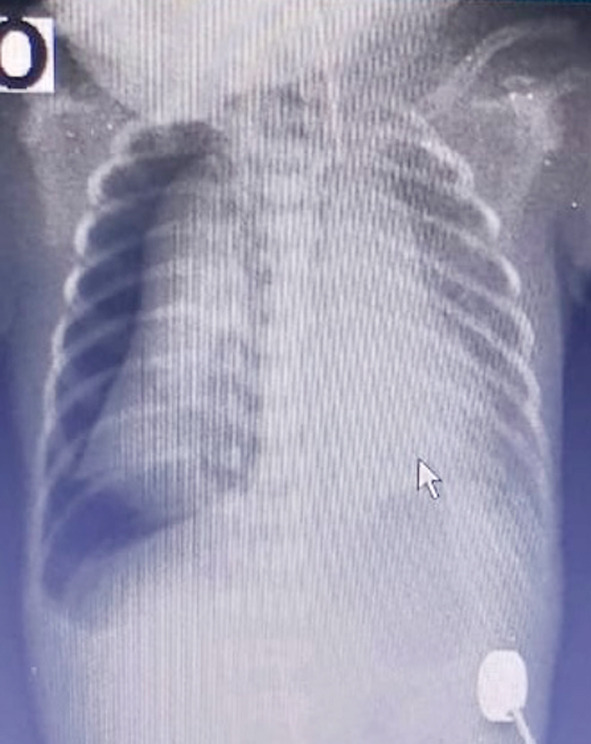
Chest X-rays showing right-sided pneumothorax.
